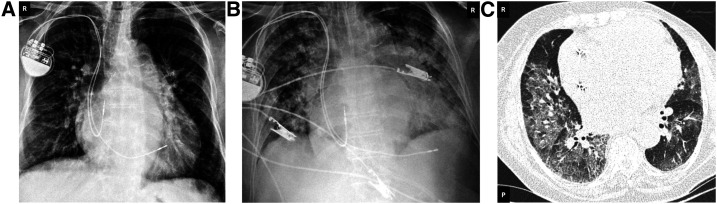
Chest X-ray and thoracic computerized tomography scan of patient #1. (A) X-ray from 2015 pre–COVID-19 hospitalization showing the marked cardiomegaly, (B) X-ray from June 2020, and (C) tomography from June 2020 during COVID-19 hospitalization showing COVID-19 ground-glass opacity.
